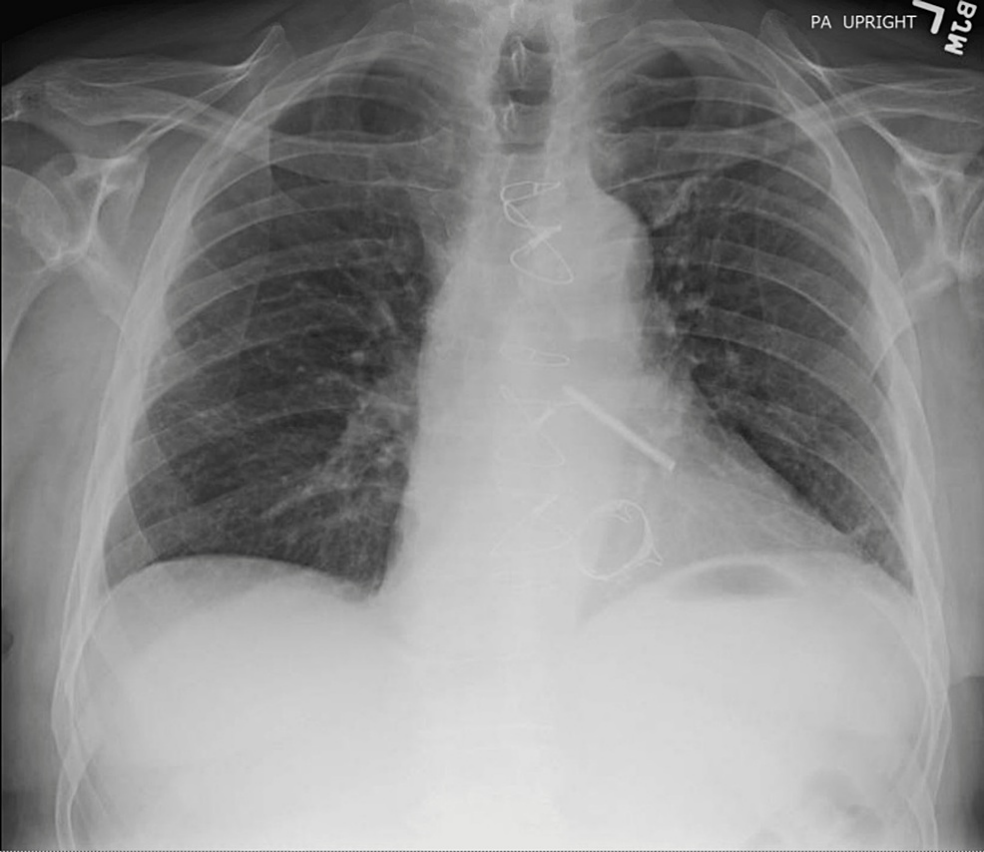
Our patient's PA chest radiograph, two weeks post-surgery, showing complete resolution of the infiltrates PA: posteroanterior
